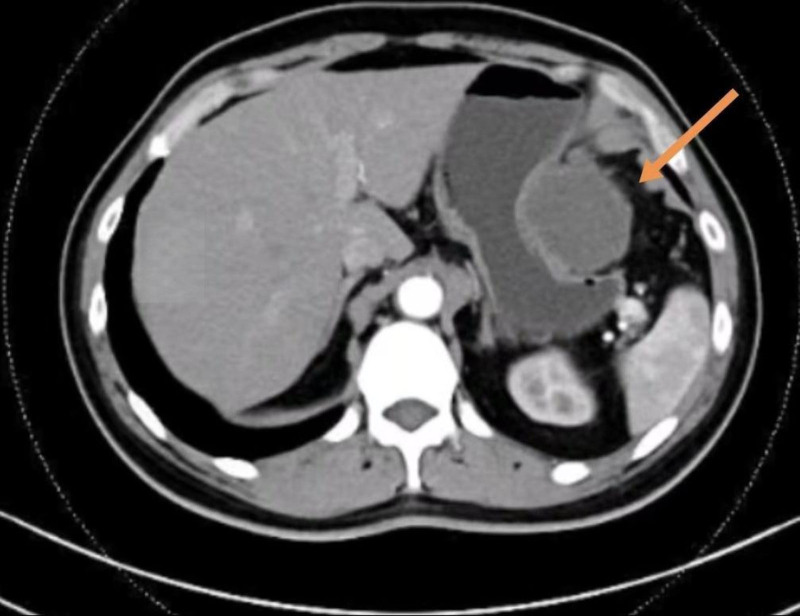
Abdominal contrast-enhanced CT showed a peripherally enhanced, multiseptated hypodense mass abutting the gastric greater curvature. CT = computed tomography.
